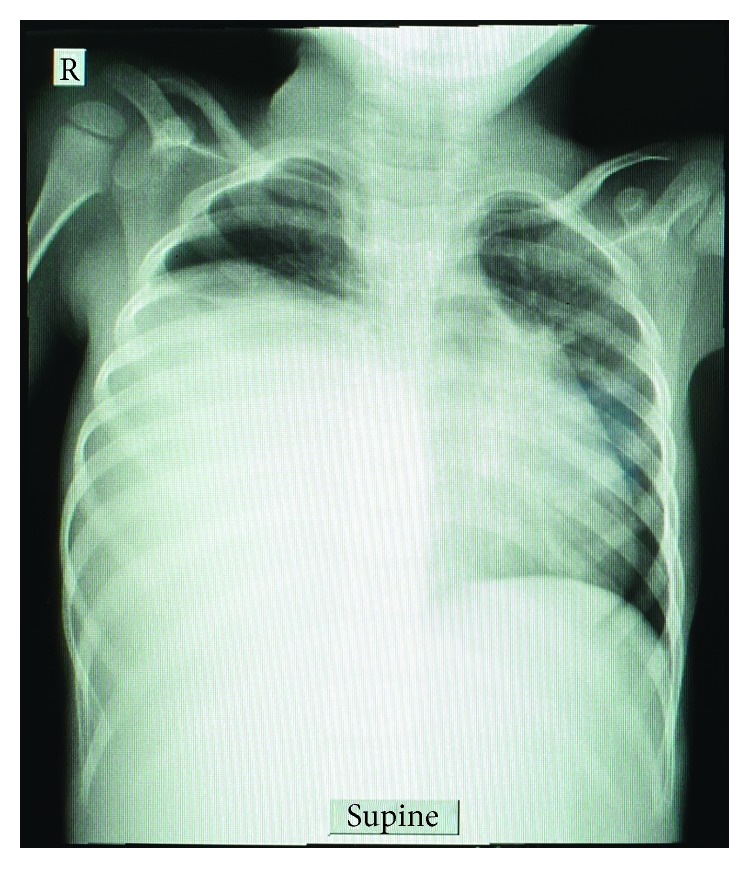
Chest X-ray showing opacification of the right hemithorax.
